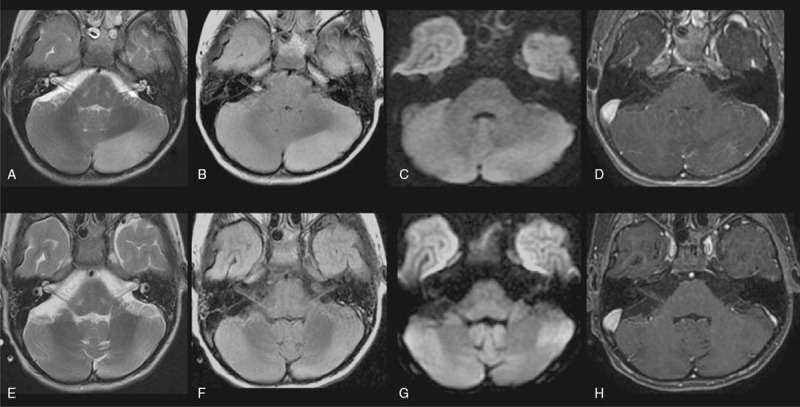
Brain MRI of a pediatric patient with autoimmune anti-GABA_A_ receptor encephalitis comorbid with an established B19 infection of the central nervous system at two timepoints. A–D. Images from the patient's first MRI scan, which occurred while he was being treated in the ICU for persistent seizures that evolved into focal SE with fluctuating consciousness. T2/FLAIR hyperintensity and mild expansion in the left cerebellar hemisphere, with some foci of contrast enhancement and an absence of restricted diffusion. E–H. Images from a follow-up scan performed almost a month later, likely showing residual post-inflammatory changes. Note that the previously observed T2/FLAIR hyperintensity and volume expansion were reduced and that there were no new lesions or areas of contrast uptake in the left cerebellar hemisphere.
